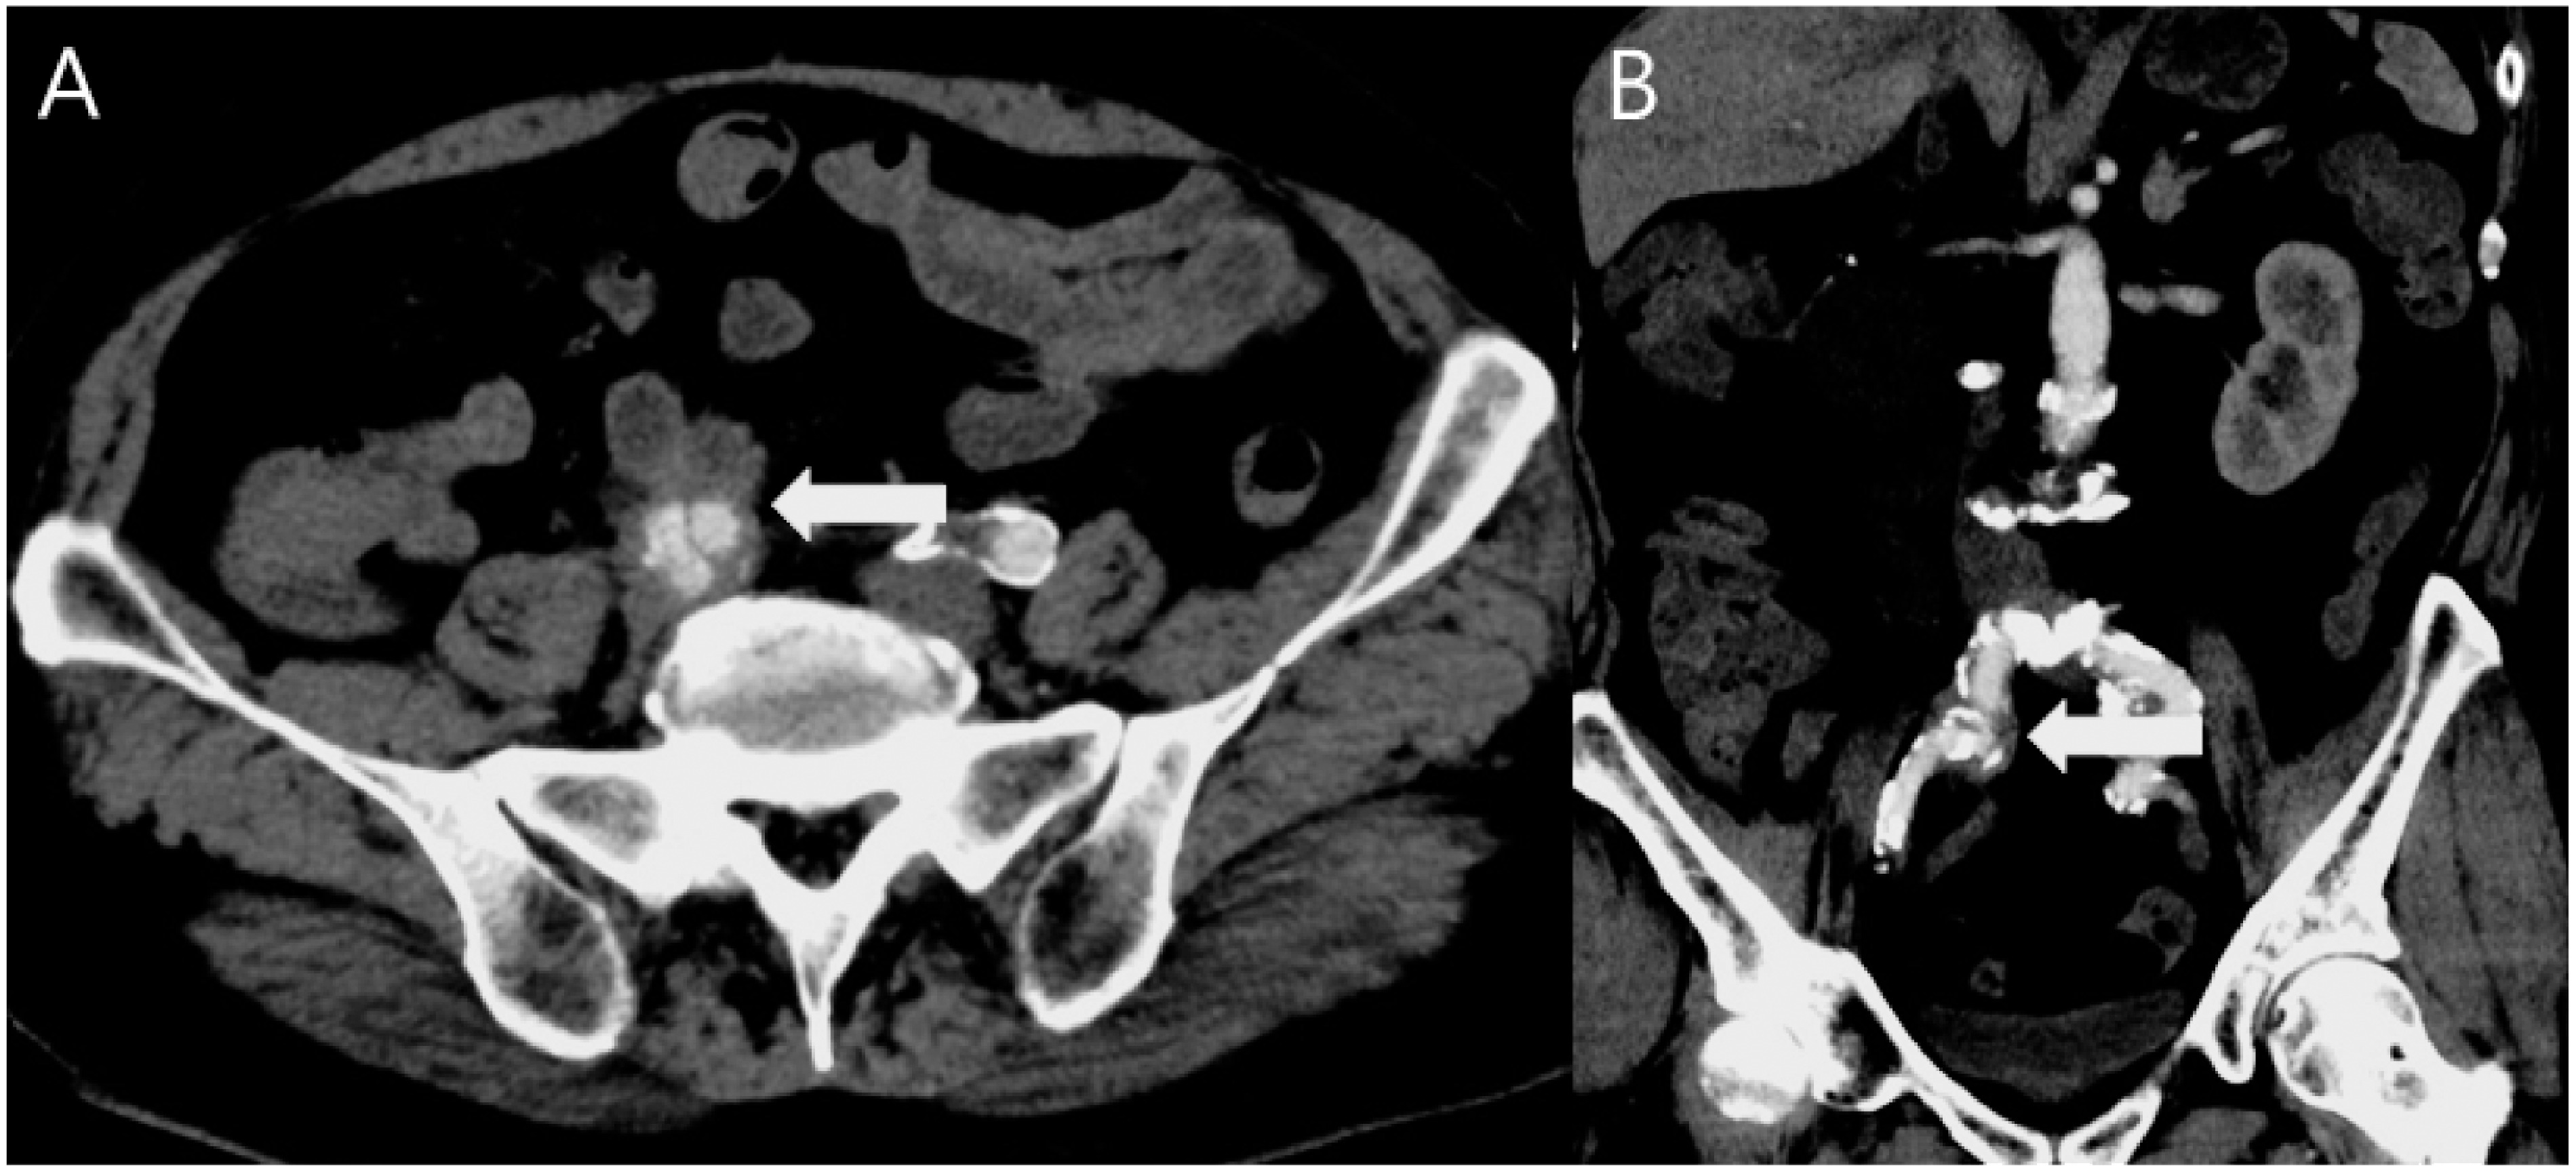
Fig. 1 Preoperative images. (A), (B) Enhanced computed tomography showed no extravasation and showed the adhesion between the iliac branch and small intestine (white arrow).
